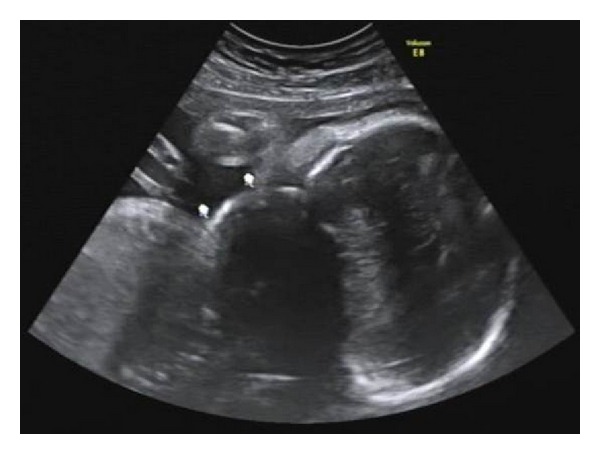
Ultrasound image at 30 weeks' gestation of a lymphangioma.
